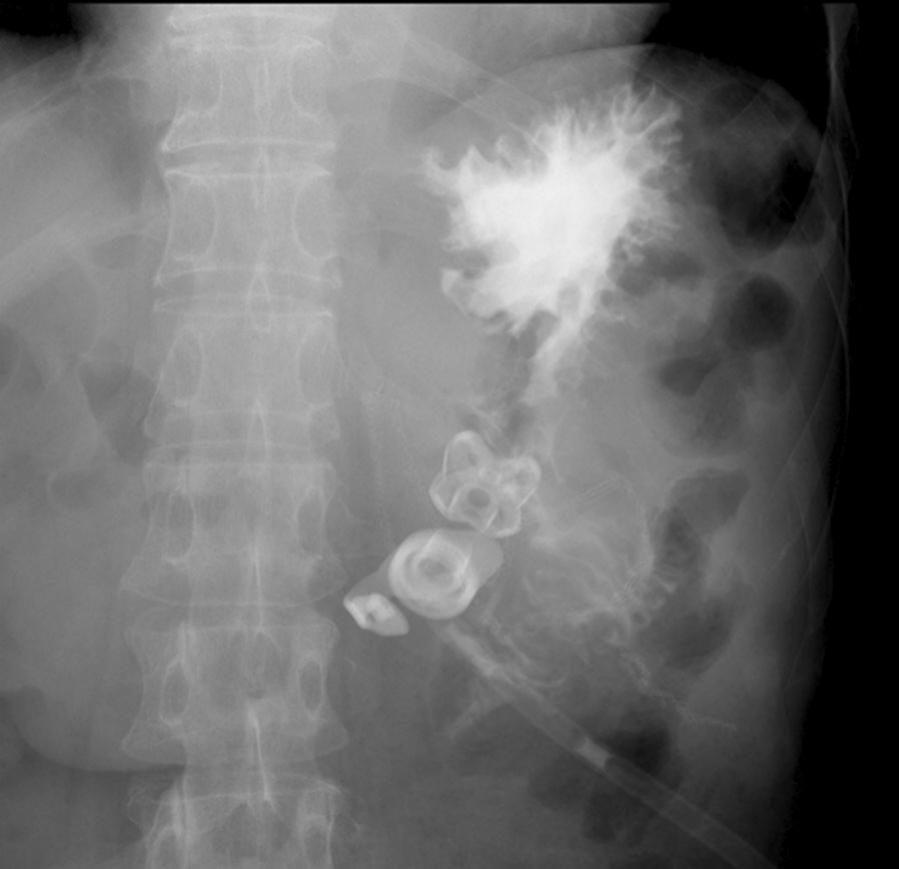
Contrast radiography findings. There was no evidence of postoperative leakage or stenosis
